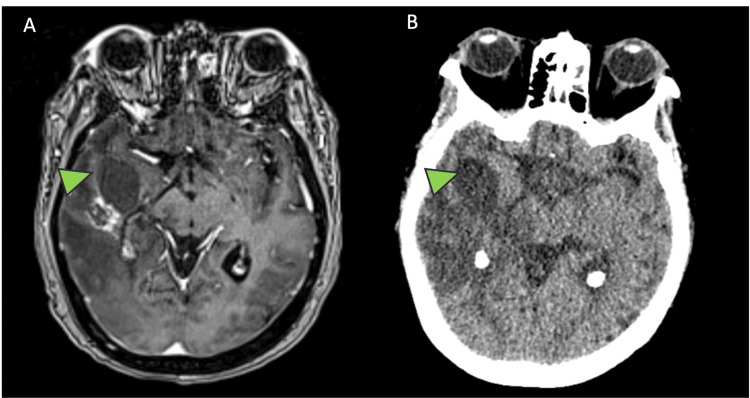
Pre-operative patient imaging. (A) Preoperative T1 MRI. (B) Preoperative CT scan showing the presence of cyst and contrast-enhancing lesion. The green arrow indicates the location of the temporal pole cyst.
